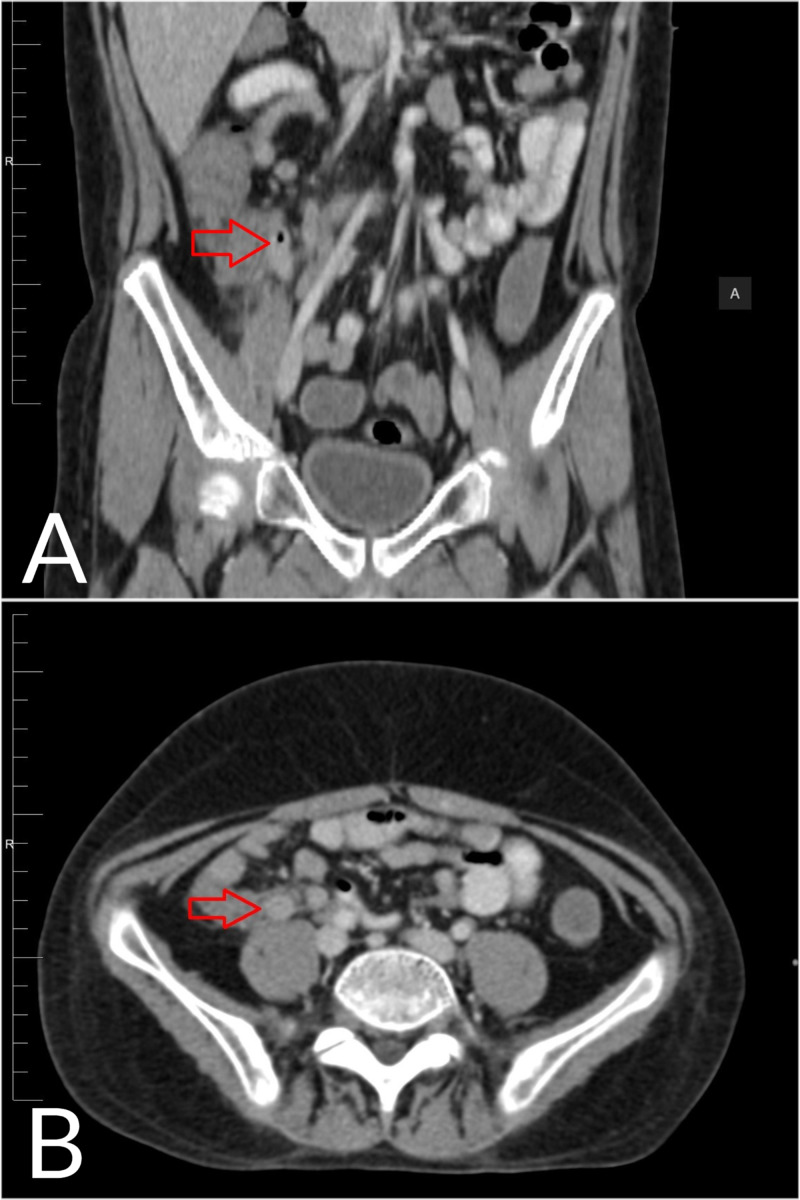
CT of the abdomen. (A) Coronal CT scan image. (B) Axial CT scan image. Images show a dilated, thick-walled appendix measuring up to 7 mm (red arrows) with peri-appendicular inflammatory fat stranding and minimal free fluid.
